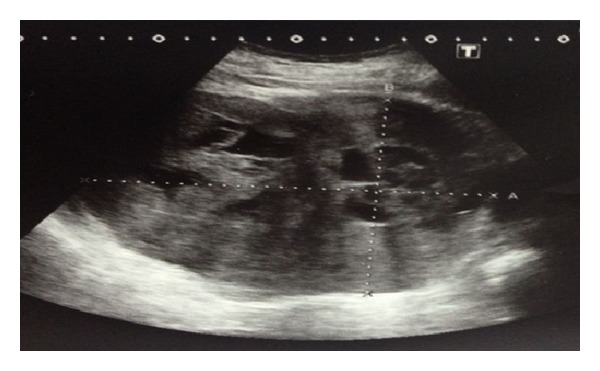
The 150 × 140 × 130 mm (RL × AP × KK) mass in left adrenal gland in abdominal ultrasonography.
